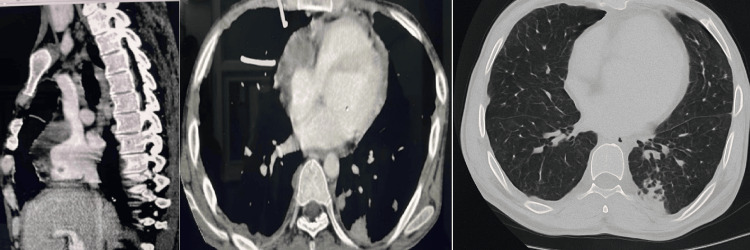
CT of the thorax showing diffuse non-enhancing thickening along the anterior wall of the right atrial (RA) and along the anterior aspect (left). Mass seen in the right atrium along with a thickened RA wall and pericardial thickening (center), with bilateral reticulonodular infiltration (right).
